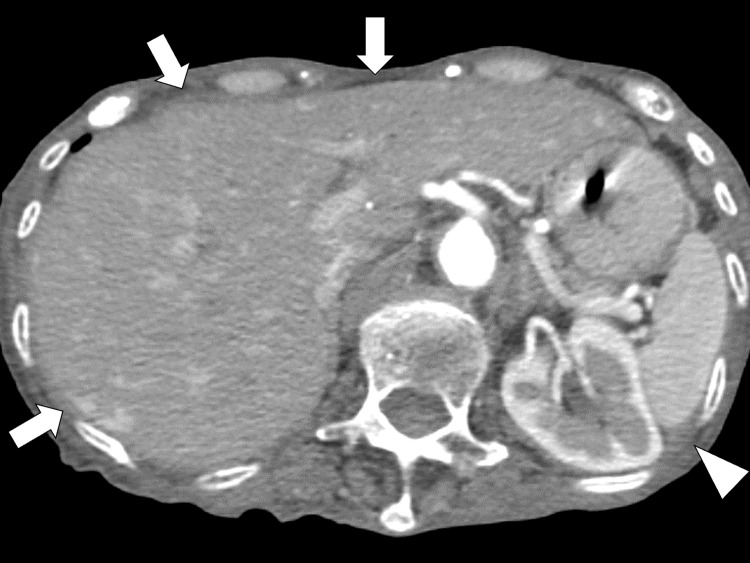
An enhanced neck-to-pelvic computed tomography scan The image is revealing pleural effusion, ascites (white arrowhead), and hepatosplenomegaly (white arrow) without prominent lymphadenopathy.
